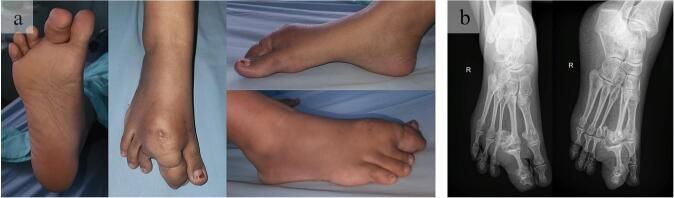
An initial (a) localized examination of the second toe of the right foot revealed a lump measuring 5x2x2 cm and (b) preoperative radiographic showing multiple exostosis on the metatarsal, proximal phalanx and distal phalanx.
